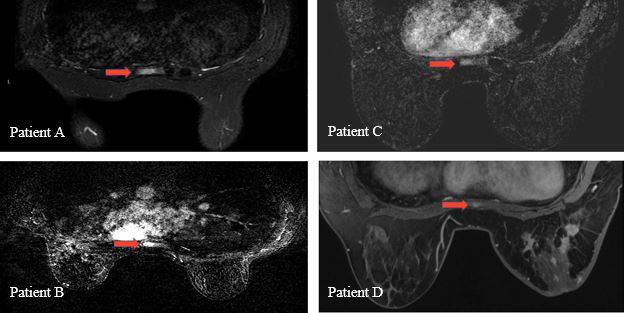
Diagnostic magnetic resonance imaging of oligometastatic sternal lesions (axial view) Red arrow indicates the location of the sternal lesion.
